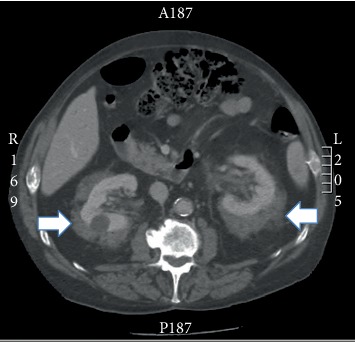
Abdominal CT scan showing symmetrical, bilateral perirenal rind-like lesions (arrows) measuring 2.5 cm in thickness, so-called hairy kidney sign.
